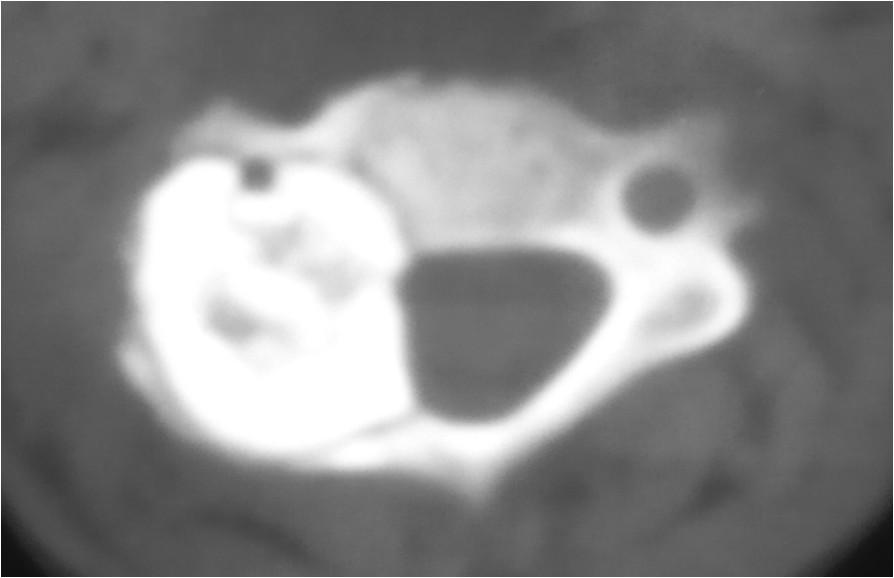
Computed tomography indicates the presence of a massive tumor from right lamina of C3 and extending to the spinal canal. The tumoral mass circumference has a sclerosis band, and the inhomogeneous matrix ossification is presented in the lesion. The canal of vertebral artery is also encroached by the lesion.
